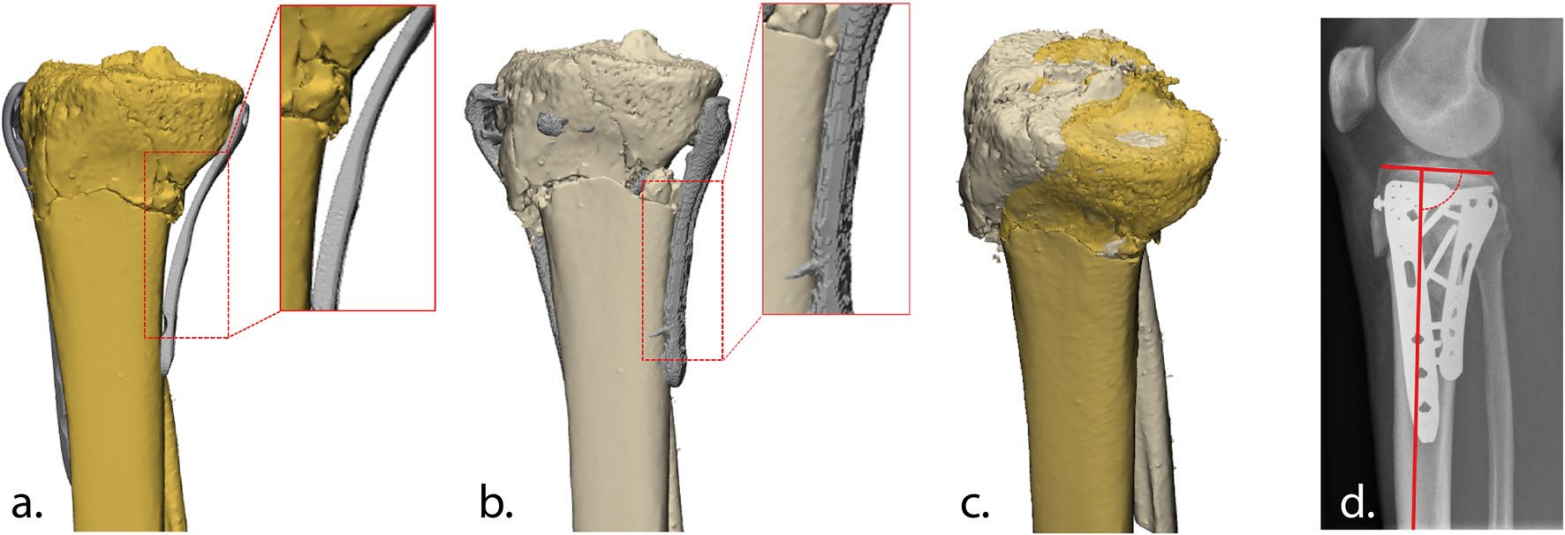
Clinical case of a female patient in her 40 s, who was treated for a Schatzker 6 tibial plateau fracture. a Surgical plan involving dual-plating including a posteromedial plate which had substantial space in between plate and bone due to suboptimal fitting. b Postoperative result: Due to uncontrolled compression on the bone with the poor-fitting posteromedial plate, the tibial shaft and the fragments are not properly aligned. c Planning (yellow) vs. postoperative result (grey). d Postoperative result on a lateral radiograph shows reduced sagittal alignment (2°) (colour figure online)
